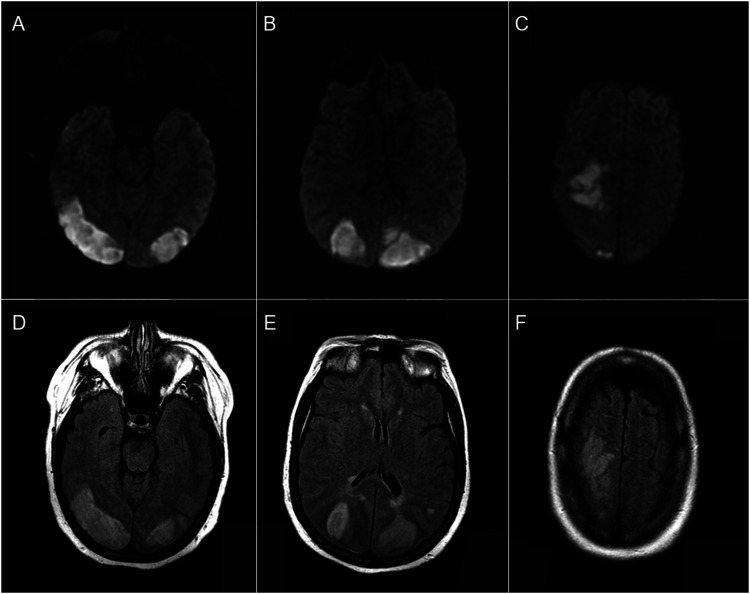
MRI showing multiple, acute bilateral temporal-occipital and right frontal acute infarctions.
